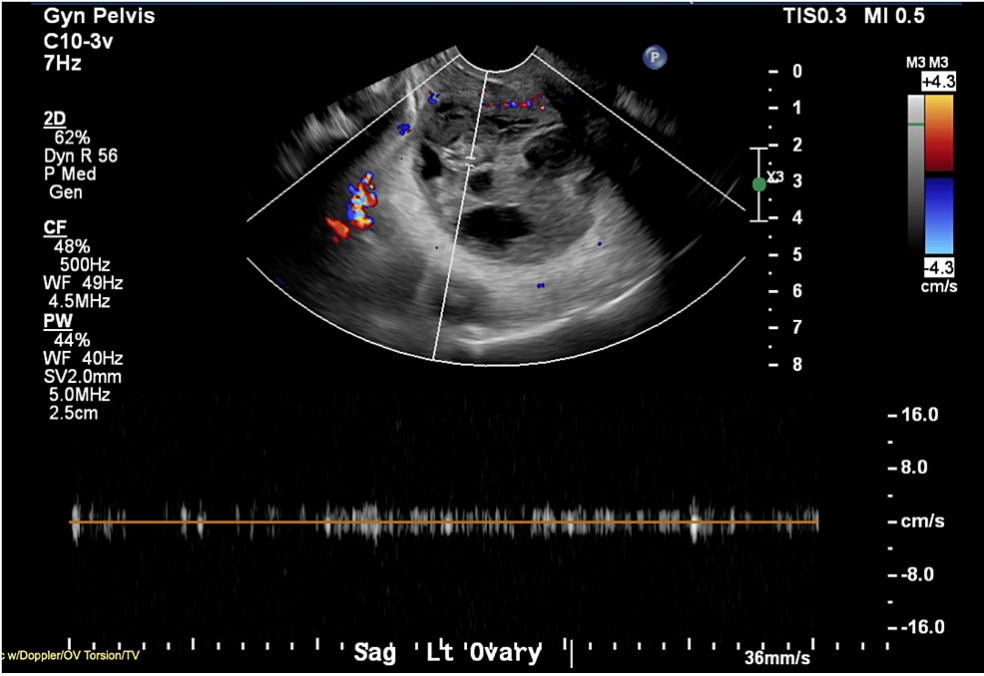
Pelvic ultrasound showing no blood flow to the left ovary
